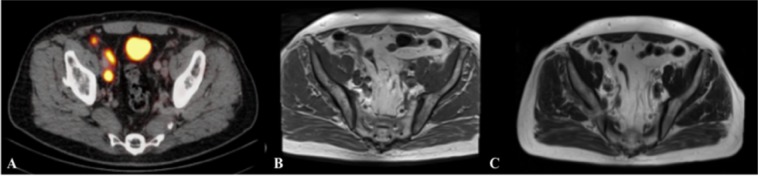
Lymph node evaluation depicted in ^68^Gallium-Prostate specific membrane antigen PET-CT and multiparametric MRI. This figure shows an example of lymph node (LN) detection using multiparametric MRI. The lymph node is visualized in corresponding axial plane slices using ^68^Gallium-Prostate specific membrane antigen PET-CT, T1 and T2 sequences of MRI presented left to right. The additional use of the T2 weighted sequence improved the detection of a LN in risk to be missed. This suspect LN is located at the right external iliac artery and is challenging to depict in the T1 sequence. (A) Lymph node depicted in ^68^Gallium-Prostate specific membrane antigen PET-CT, (B) Lymph node depicted in the T1 sequence in MRI; (C) Lymph node depicted in the T2 sequence in MRI.
